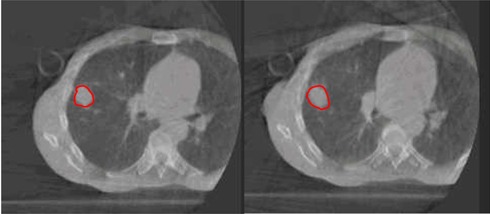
An example of GTV (red line) volume difference between the two CBCT scans for Patient 3: an image taken before SBRT (left) and an image taken after completing the 3rd fraction of SBRT (right).
